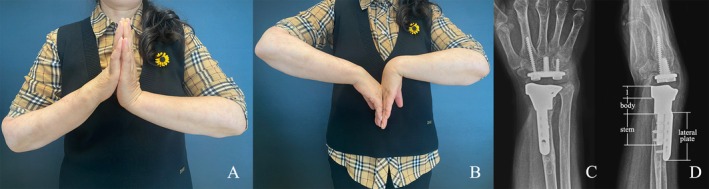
Postoperative range of motion and radiography of Patient 4 after 6 months. (A, B) Range of wrist flexion and extension. (C, D) The radiographic appearance of the prosthesis at the final follow‐up (D1) The articular surface structure.
